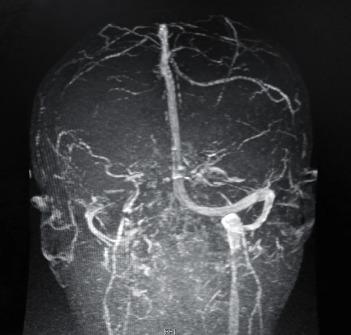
MRI and MRV brain showing no flow within the left transverse and sigmoid sinuses and also no flow within the left internal jugular vein.
